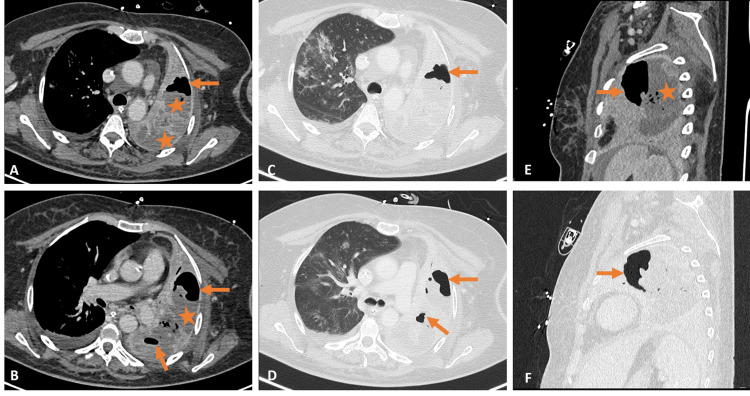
Chest computed tomography performed 17 days after hospitalization Axial enhanced computed tomography images at the apex (A) and mid-lung (B) continue to demonstrate heterogeneously enhancing left lung consolidation (asterisks) and increasing abscesses (arrows). Axial enhanced computed tomography images in lung windows at the apex (C) and mid-lung (D) and sagittal reformatted images in mediastinal (E) and lung (F) windows depict the same findings.
